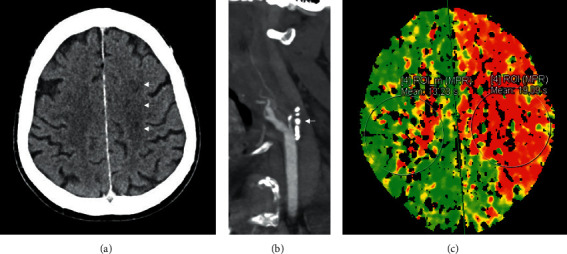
(a) Noncontrast CT: small border zone infarcts (white arrows) ASPECTS 10. (b) CT angiography: occlusion of the left ICA caused by a preexisting atherosclerotic stenosis (white arrow). (c) Time-to-peak map of CT perfusion: delay in maximum attenuation of 5.7 seconds.
